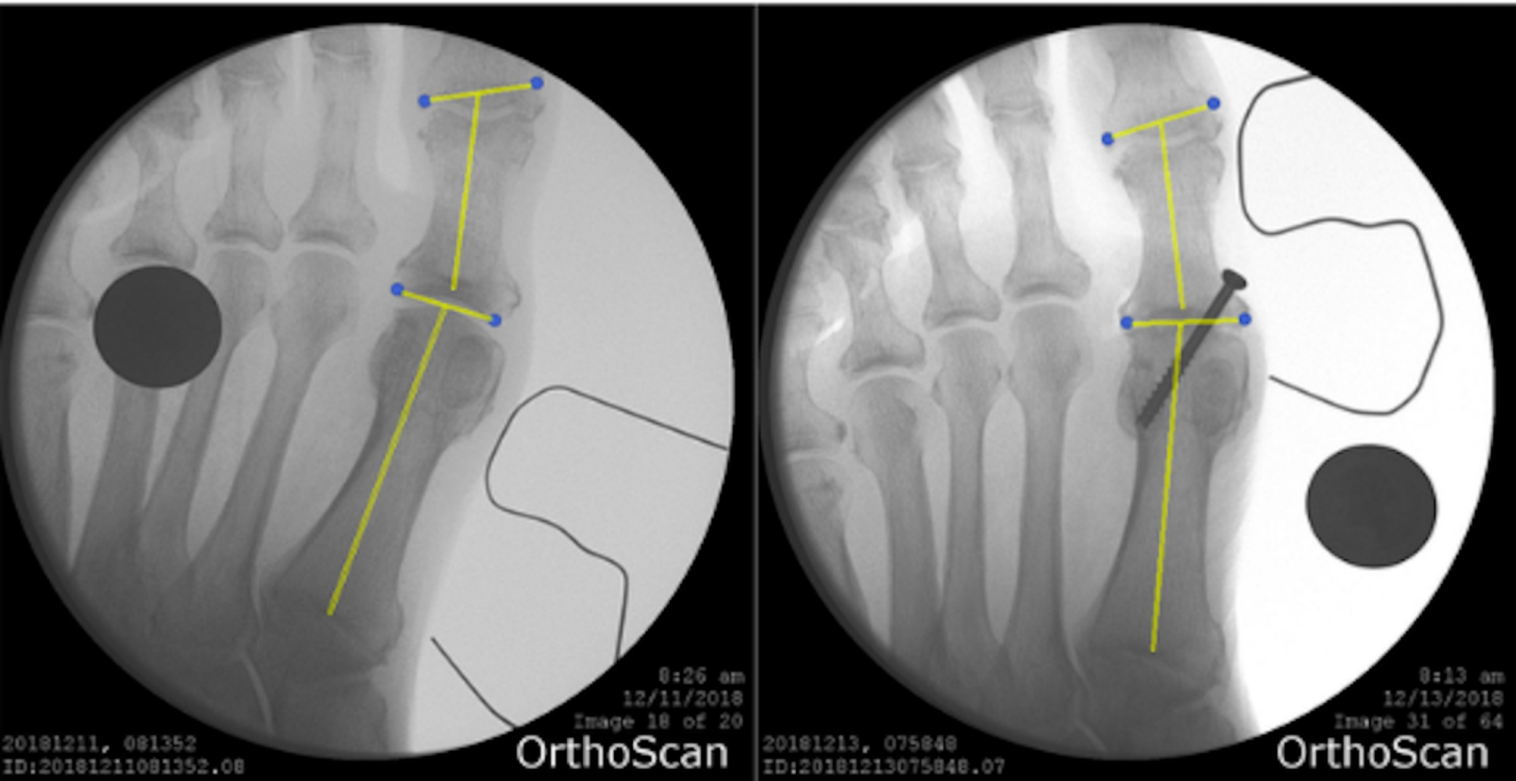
First Ray Plain Film Pre- and post-operative measurement of the first ray length using ImageJ software analysis of fluoroscopic images.
